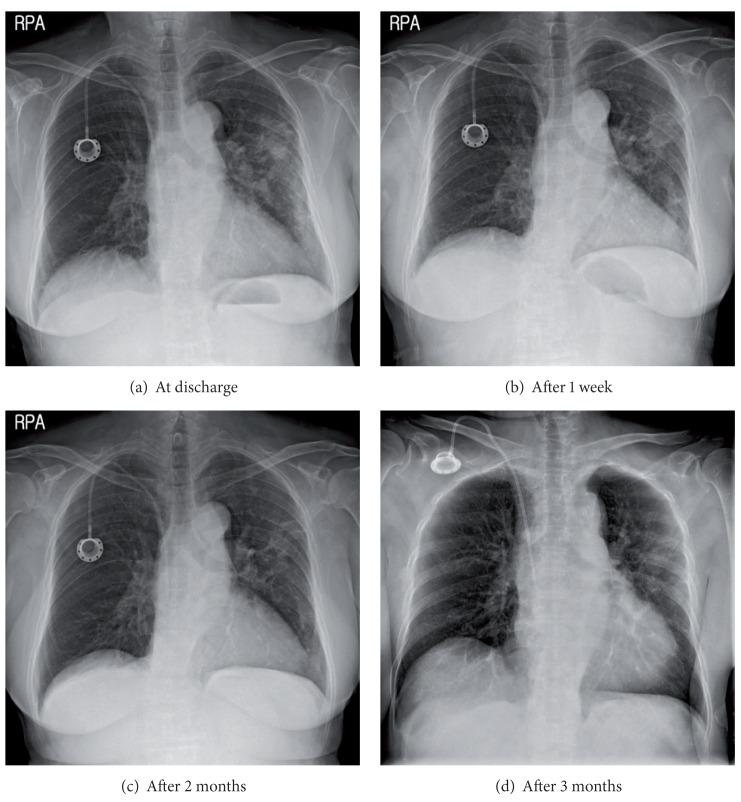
Resolution of chest X-ray after treatment for sarcoidosis.
